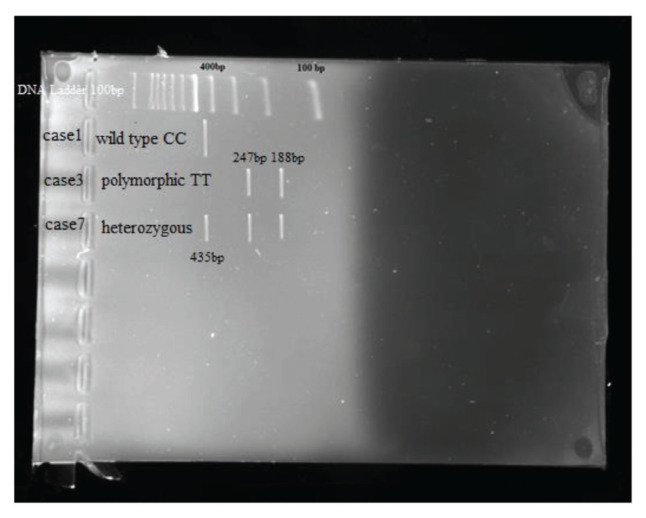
Samples of MMP-9 PCR-RFLP (435 bp) CC genotype with one fragment band, TT genotype with two fragments are polymorphic and CT genotype with three fragments band are heterozygous
